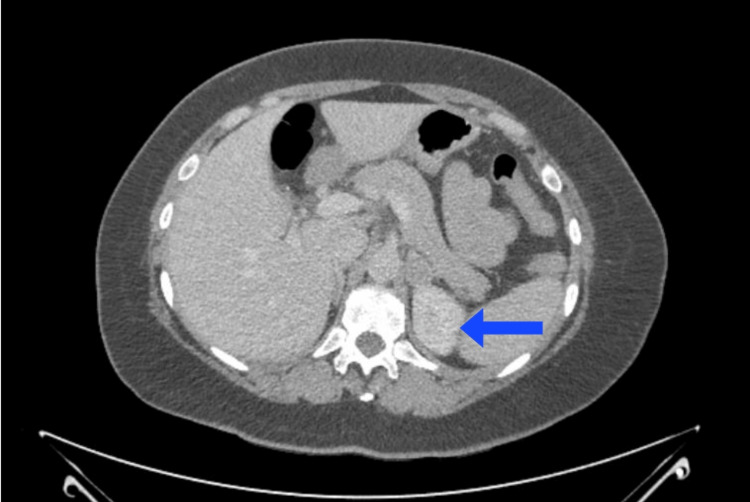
Abdominal computed tomography (CT) scan. Arrow points to a 3 cm pheochromocytoma deep in the left retroperitoneal flank region of a 45-year-old man who presented with a history of episodic headaches, palpitations, and sweating for the past six months.
